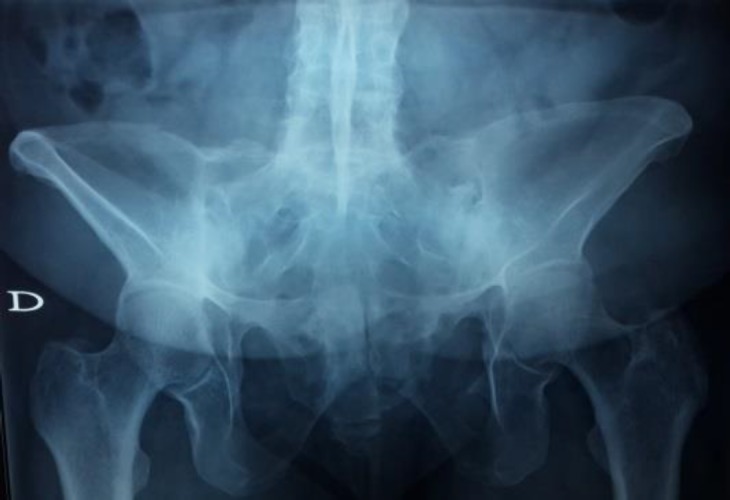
Pelvic x-rays showed a bilateral sacroiiitis grade 4
